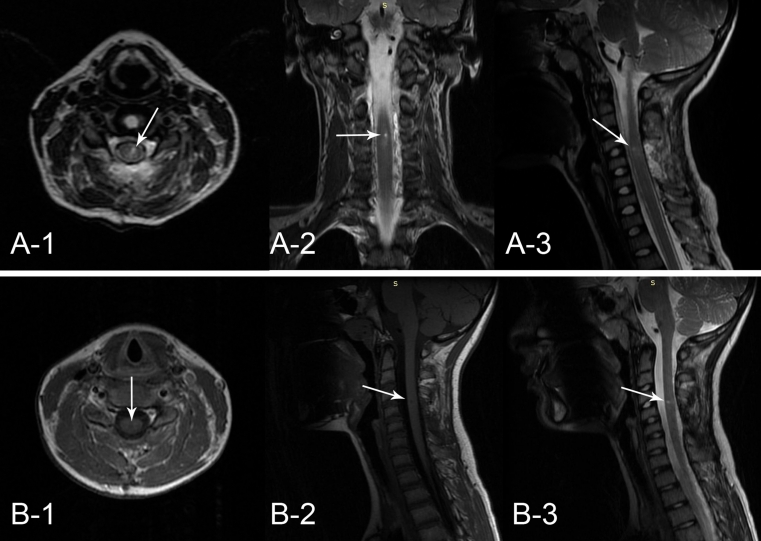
Postoperative control MRI scans. a early postoperative control; b control scans after 6 months from surgery. *1*,*2*,*3* different aspects. *Arrows* indicate the site of the lesion
